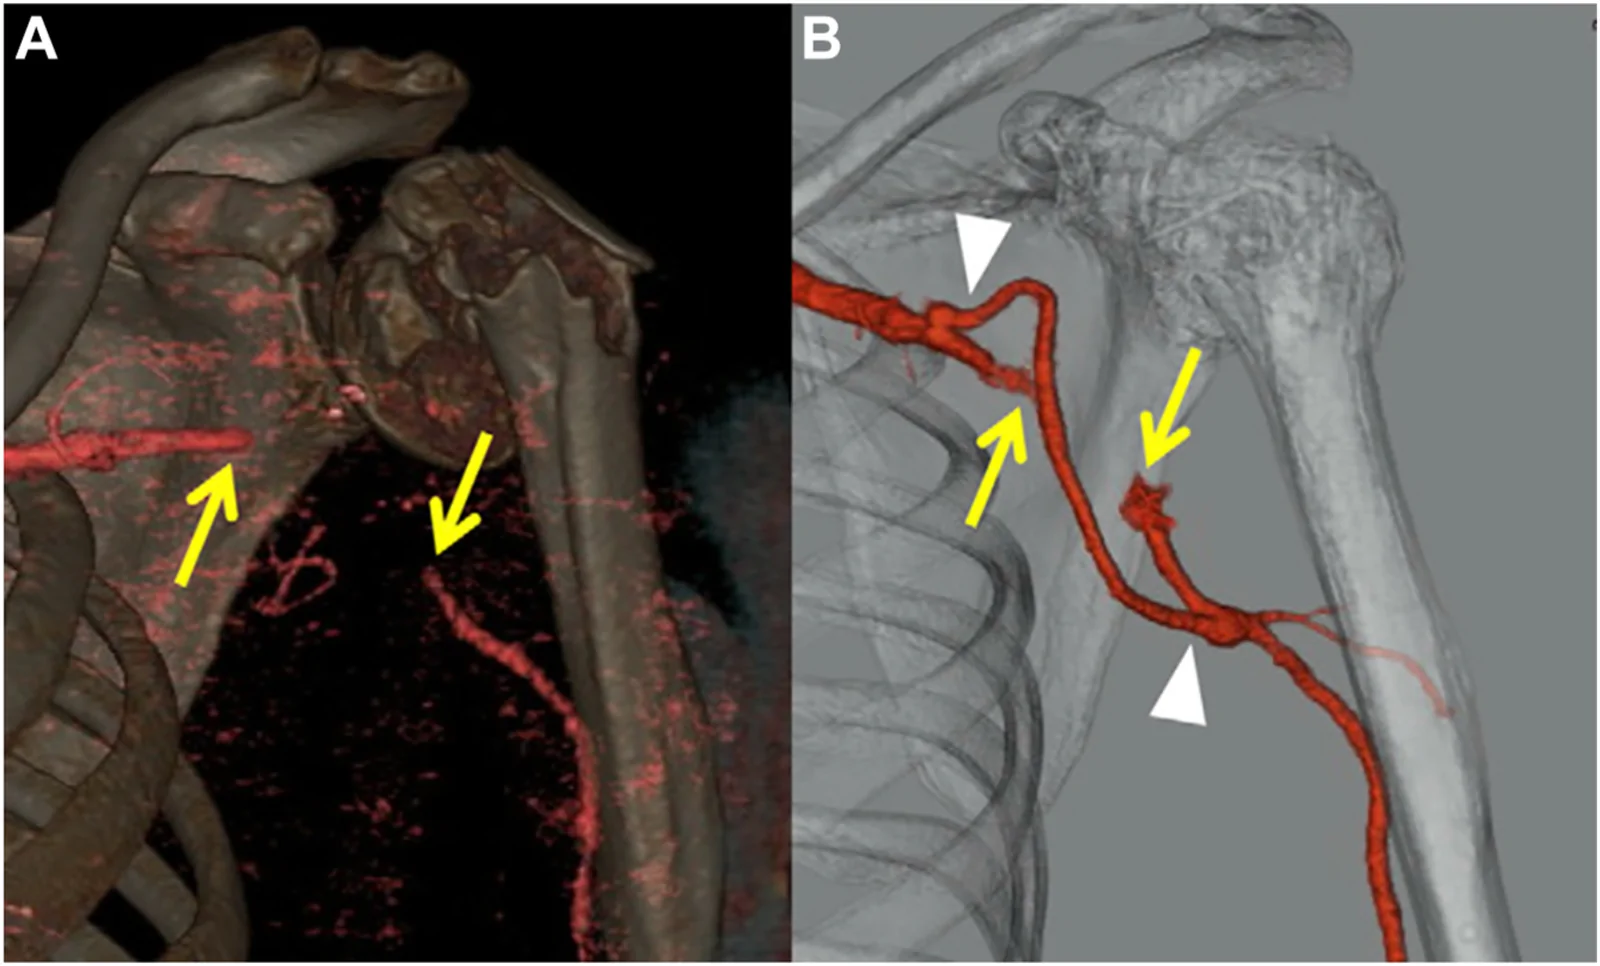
(A) Adapted from Mitsuzawa et al, 2024. Three-dimensional CTA demonstrating axillary artery disruption (*yellowarrows*) in a 69-year-old male who sustained a low-energy fall while intoxicated. The patient presented with severe shoulder pain, a cold hand with weak radial pulse and motor–sensory deficits in the radial and median nerve distributions. Radiographs and CT imaging revealed a three-part PHF. CTA showed a contrast defect of the axillary artery at the third segment, while distal vessel opacification was preserved through collateral circulation. (B) Post-operative CTA demonstrating restoration of axillary artery continuity following surgical repair. Five days after the injury, the patient underwent hemiarthroplasty (HA), after which the injured segment of the axillary artery was bypassed using a vein graft with an end-to-side anastomosis (*white arrowheads*), and neurolysis of the brachial plexus was performed.^29^*PHF*, proximal humerus fracture; *CTA*, computed tomography angiography.
